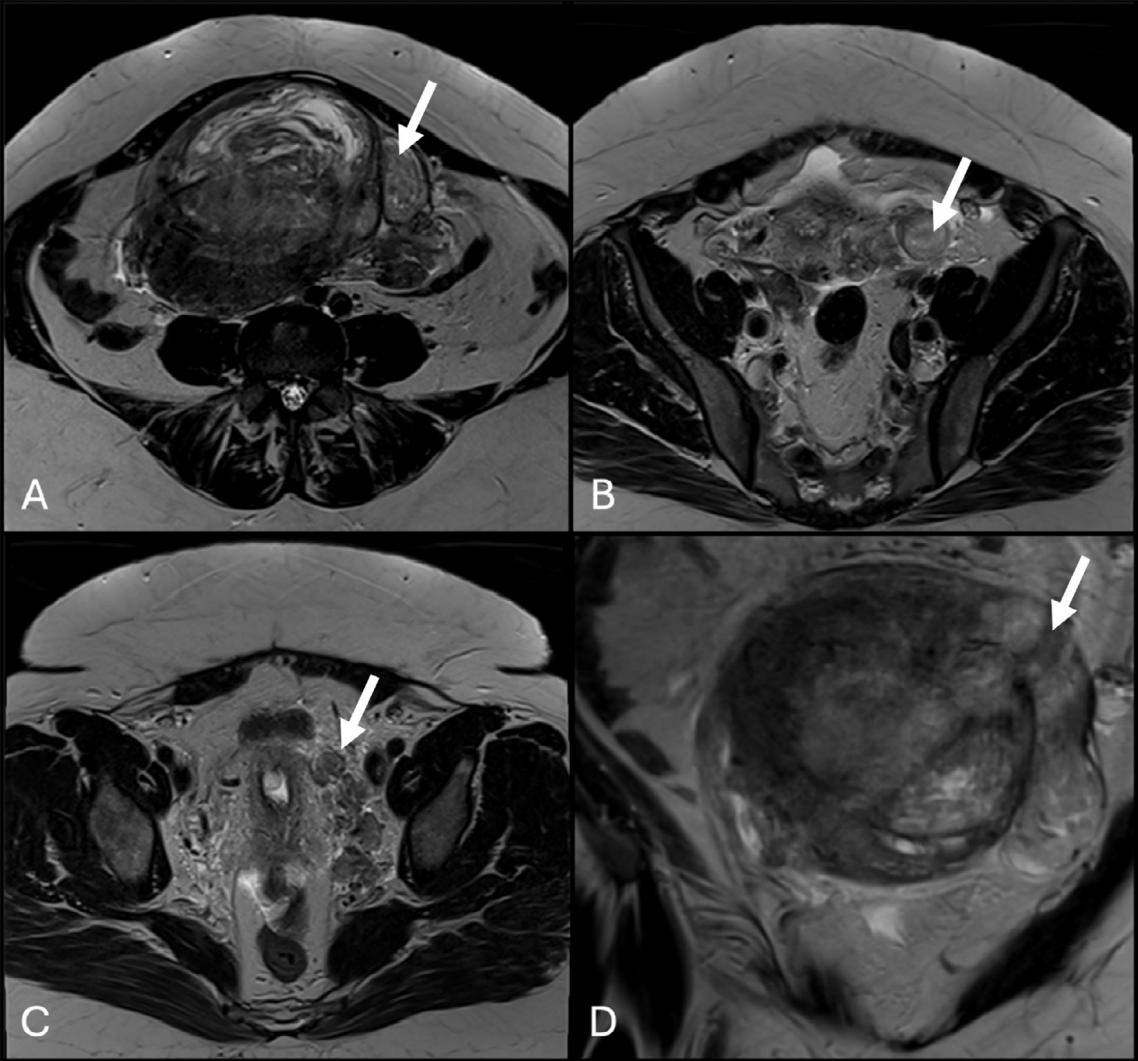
Axial (A–C) and coronal (D) T2-weighted images showing a large heterogeneous myometrial mass with lobulated contours and serpiginous extensions (arrows). Axial images (A–C) represent different levels of the tumor from superior to inferior.
